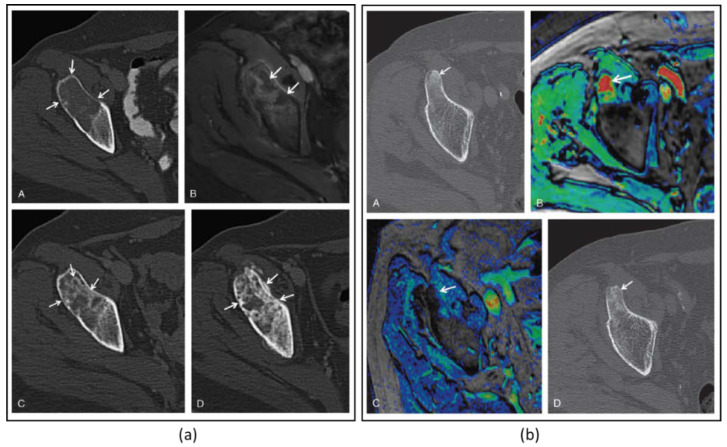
(a) Images of a 64-year-old woman with iliac bone metastasis from breast cancer. A: Axial CT image shows a wide lytic lesion located in the right anterior superior iliac spine (arrows) with evidence of focal cortical erosion, causing severe pain (pain severity score, 10). B: Axial contrast enhanced T1-weighted MRI (CE-MRI) after the MR-HIFU treatment shows the presence of some small areas of NPV (arrowheads) inside the lesion and at the periosteal margin. C: At the 2-month follow-up, axial CT identified the presence of some focal areas of de novo mineralization inside the treated tissue with partial restoration of cortical borders (arrowheads). D: At 3 months after the treatment, the lesion showed further denovo remineralization of the ablated tissue (arrowheads). On the basis of MDA criteria, this patient was classified as a partial responder; pain severity score dropped to 0 throughout the follow-up period. (b) Axial CT image of a 63-year-old man with primary prostate cancer. A: The arrowhead shows a metastasis located in the right anterior-superior iliac spine with a hyperdense pathologic nodular nucleus. B: The color-coded T1-weighted CE-MRI acquired reveals intense enhancement of the pathologic area (arrow). At 3 months after the MR-HIFU treatment, CE-MRI (C) showed a marked reduction of the lesion enhancement (arrow). D: The axial CT image acquired the same day reveals increased density in the treated area and the disappearance of the nodular pathologic tissue (arrow). On the basis of the MDA criteria, this patient was classified as a complete responder. Figures adapted and reprinted with permission from Napoli A, Anzidei M, Marincola BC, Brachettti G, Ciolina F, Cartocci G, Marsecano C, Zaccagna F, Marchetti L, Cortesi E, and Catalano C, Primary Pain Palliation and Local Tumor Control in Bone Metastases Treated With Magnetic Resonance-Guided Focused Ultrasound, Investigative Radiology, 48, 6, 351-358, https://journals.lww.com/investigativeradiology/Abstract/2013/06000/Primary_Pain_Palliation_and_Local_Tumor_Control_in.1.aspx accessed on 21 December 2022 [30].
